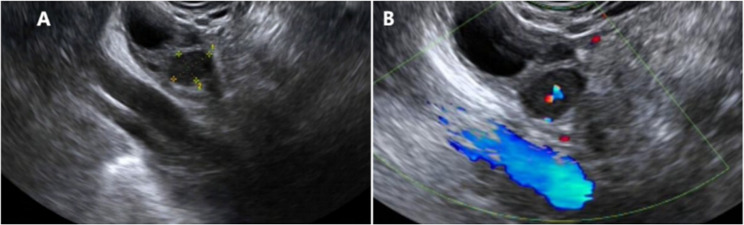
A 27-year-old patient, with right lower abdominal pain for 2 weeks. A Ultrasound revealed a rounded weakechogenicity in the right ovary, with clear and regular borders. B regular striped blood flow signals were observedboth in the periphery and inside of the mass the postoperative pathological examination confirmed cellular fibroma
